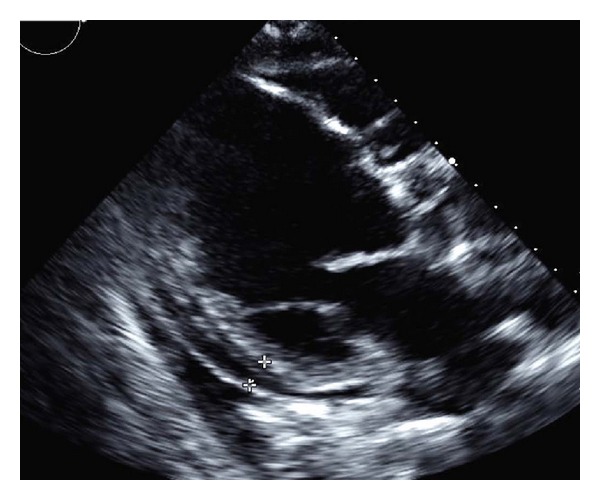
Transthoracic echocardiogram showing a moderate-sized pericardial effusion (marked).
